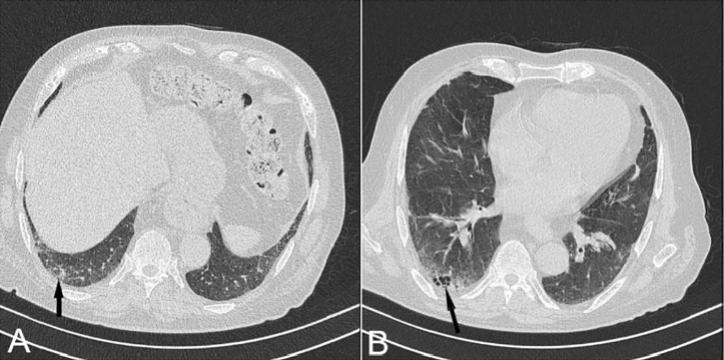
DIP: Non-contrast axial chest CT scan of 54-year-old man, smoker with progressive dyspnea and dry cough showed subpleural patches of GGO.
